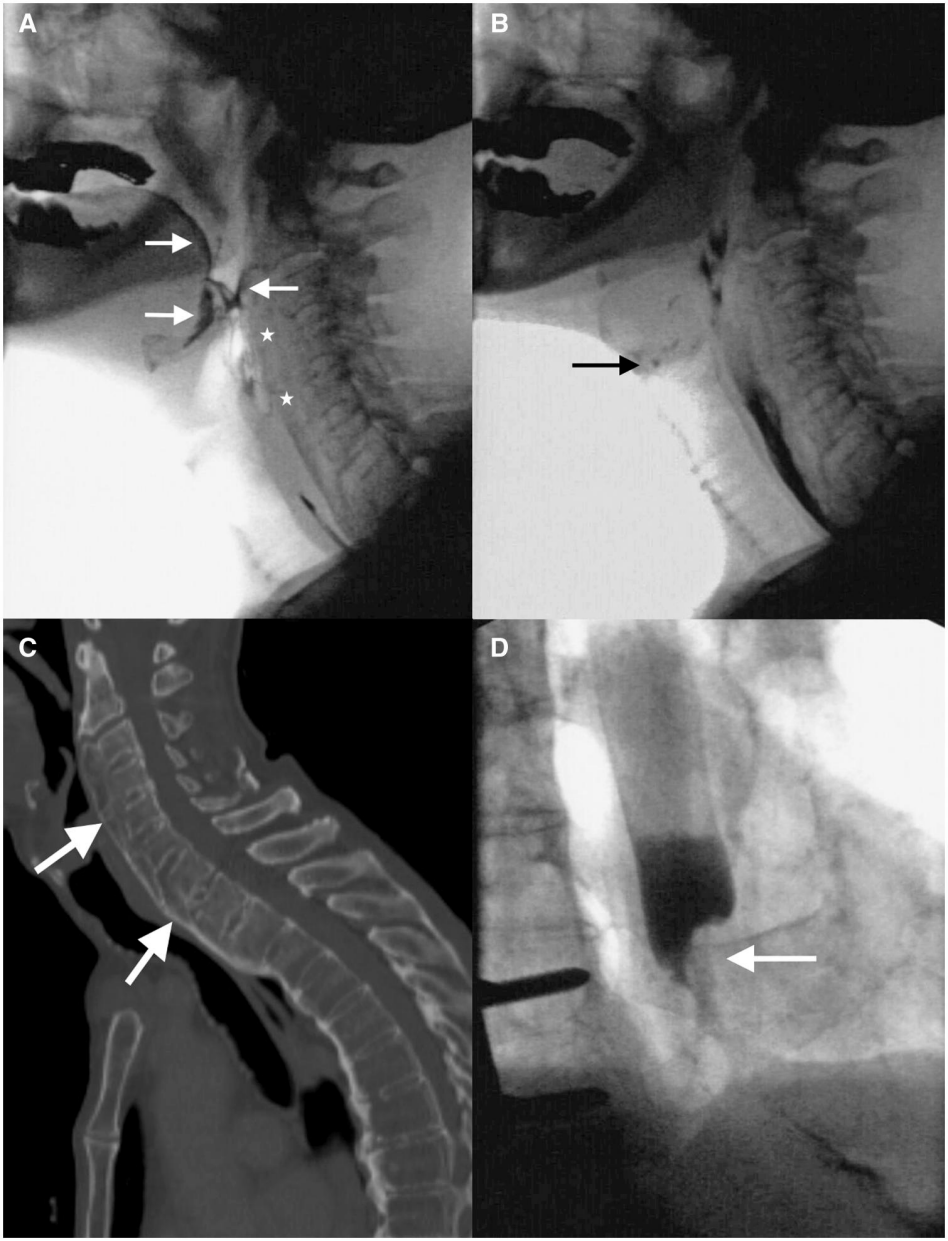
Combined pharyngeal and oesophageal pathology. (A) 78-year-old patient with dysphagia and globus sensation in the cervical region for 6 weeks. Fluoroscopy revealed diffuse idiopathic skeletal hyperostosis (asterisk) with postdeglutitive pharyngeal residues (arrows) due to pharyngeal weakness and (B) minimal deep penetration (arrow), which is a possible explanation for his symptoms. (C) CT shows the extent of ossification of the anterior longitudinal ligament (arrows). (D) Oesophageal assessment revealed irregular, asymmetric stenosis in the distal oesophagus (arrow) with transient delayed bolus passage. Subsequent endoscopy confirmed oesophageal carcinoma.
